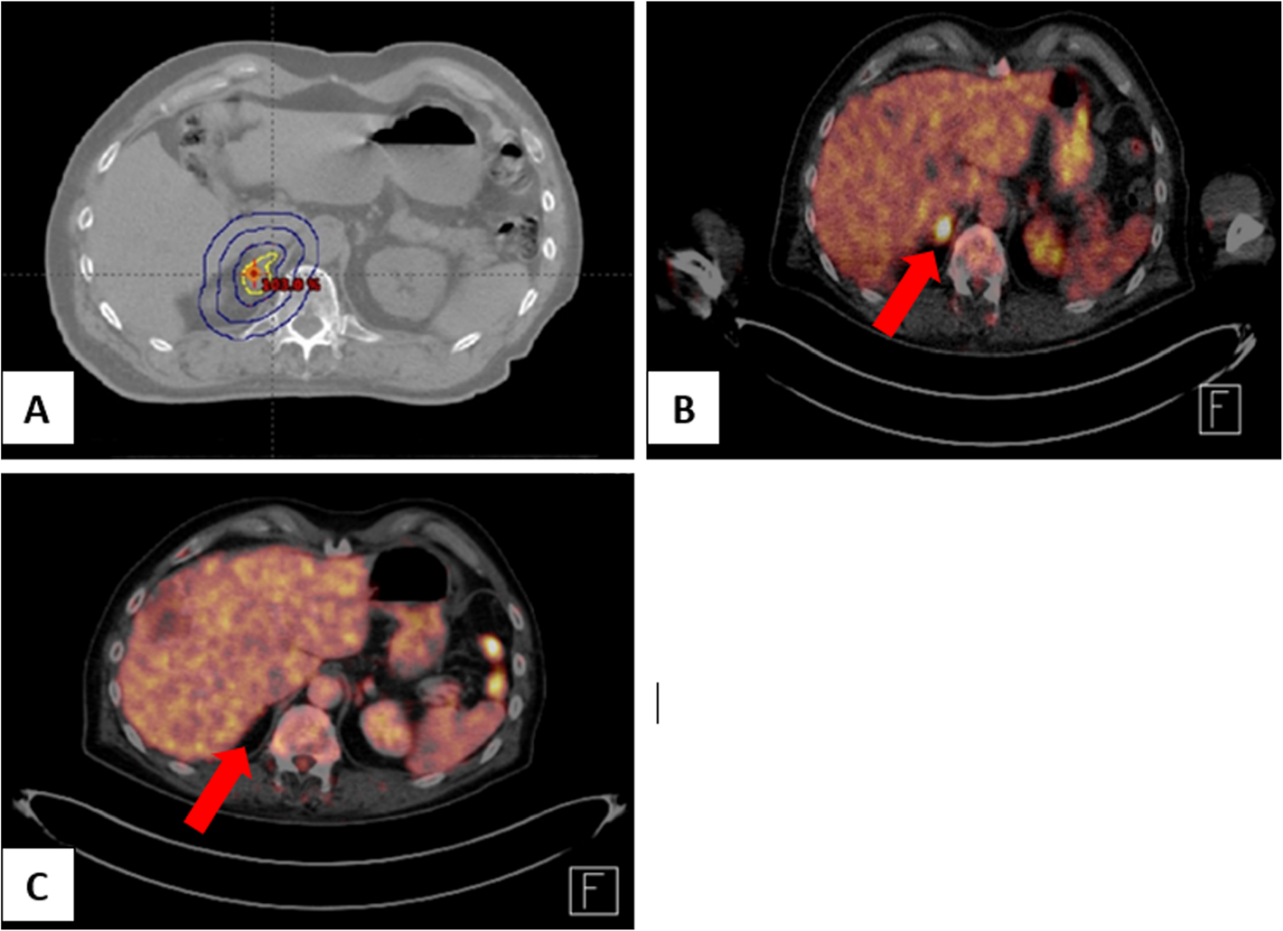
Example of a patient with malignant melanoma, who successfully underwent SBRT of adrenal gland metastasis. a CT scan and treatment plan before RT with 40 Gy in 5 fractions. b PET scan of the adrenal lesion before treatment (marked with red arrow). c PET scan four weeks after SBRT
